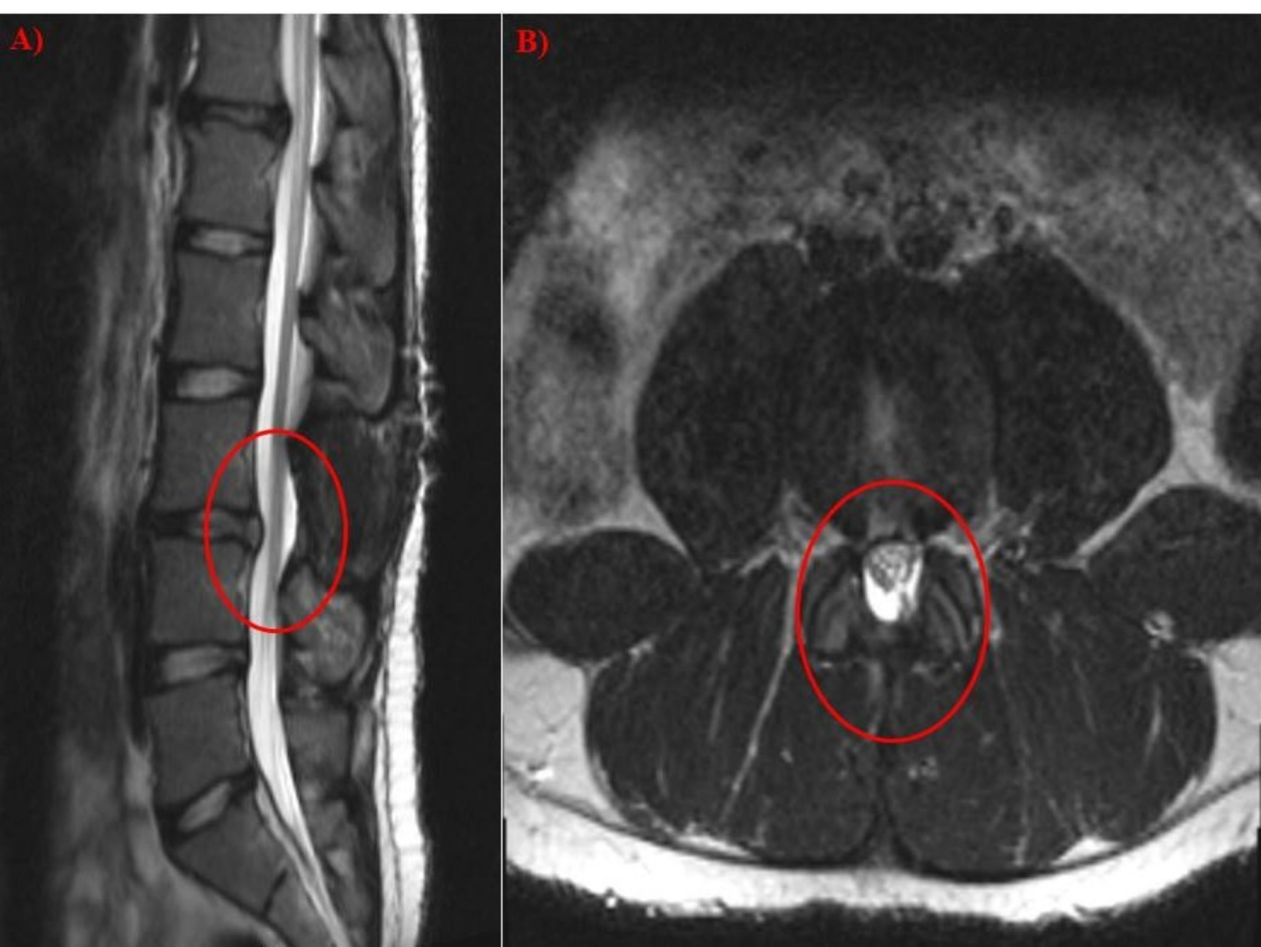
Post-operative MRI (A) Sagittal MRI T2 showing a laminectomy and removal of spinous process at the L3 level with removal of disc and restoration of the spinal canal space. (B) Axial T2 MRI of the L3-L4 disc space demonstrating the laminectomy and restoration of the thecal sack. MRI: Magnetic resonance imaging
